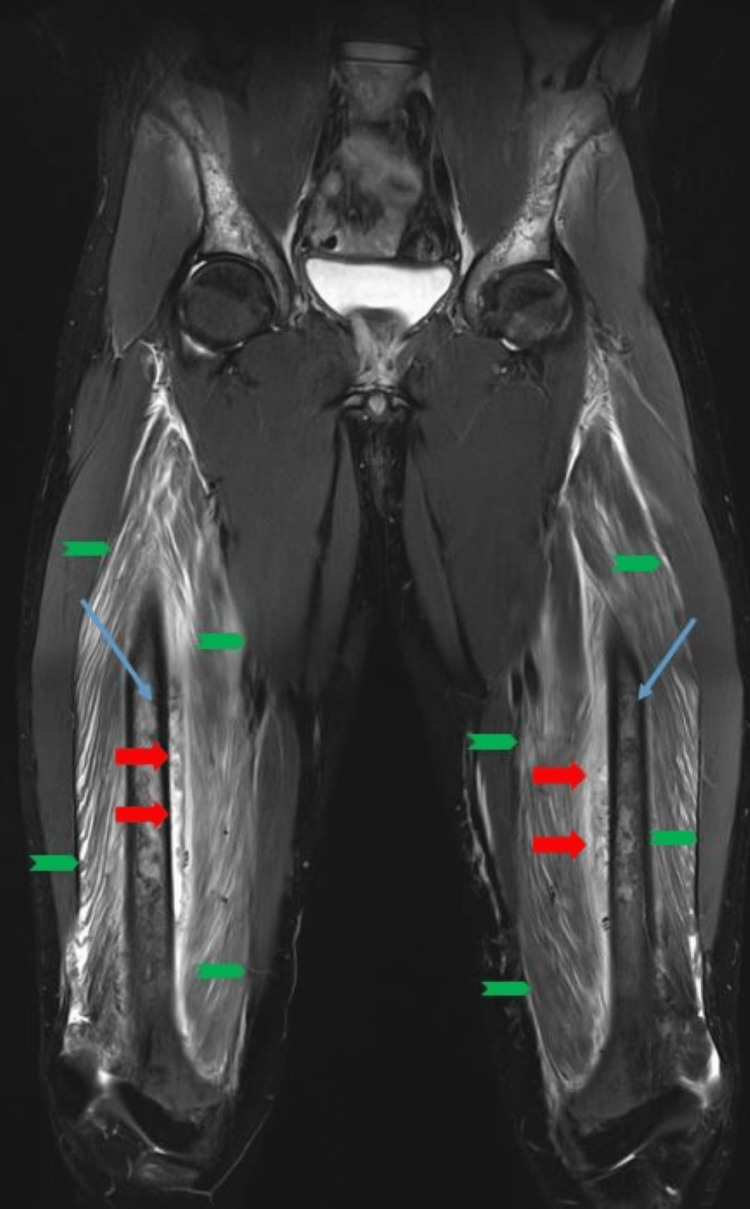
Day 6 coronal MRI scan A coronal T2 short inversion time inversion (STIR) image (Siemens 3T MRI scanner; Munich, Germany) was taken of the lower limbs and pelvis from approximately 5 cm above the iliac crest to approximately 10 cm below the knee joints on Day 6 after hospital admission. The image showed focal, high signal intensity muscle edema of the anterior compartment of the thigh, almost exclusively limited to the vastus intermedius muscle with minimal affection of the vastus medialis and biceps femoris (green arrows). Multiple bone infarcts (blue arrow) were seen in both the femur diaphysis as well as the iliac bones on both sides. There was also a small amount of muscle edema seen in the muscles around the iliac bones. In addition, there was significant periosteal lifting with associated fluid signal (red arrows) medially along both femur shafts where the infarcts had occurred in the thighs.
